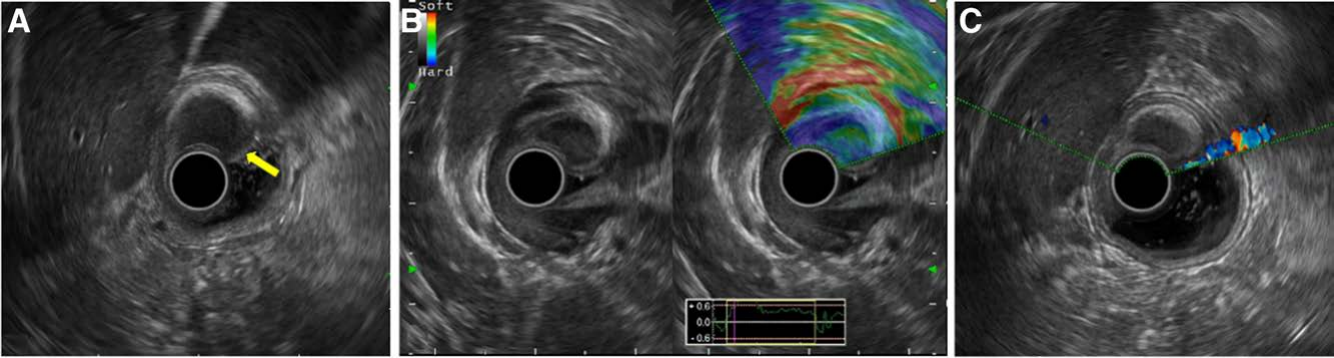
EUS showed a hypoechoic medium hard texture mass originating in the submucosal layer (A, B), originating in the submucosal layer, and CDFI showed no blood vessels inside the lesion (C). CDFI = color Doppler flow imaging, EUS = endoscopic ultrasonography.
